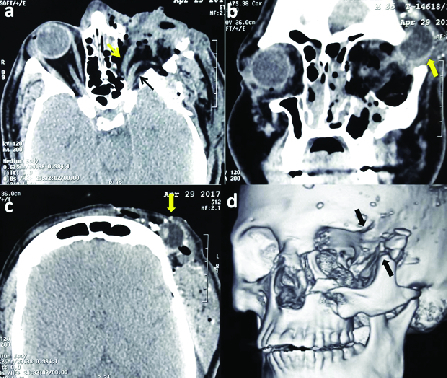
Computed tomography images of the orbit, brain, and 3D face reconstruction.
